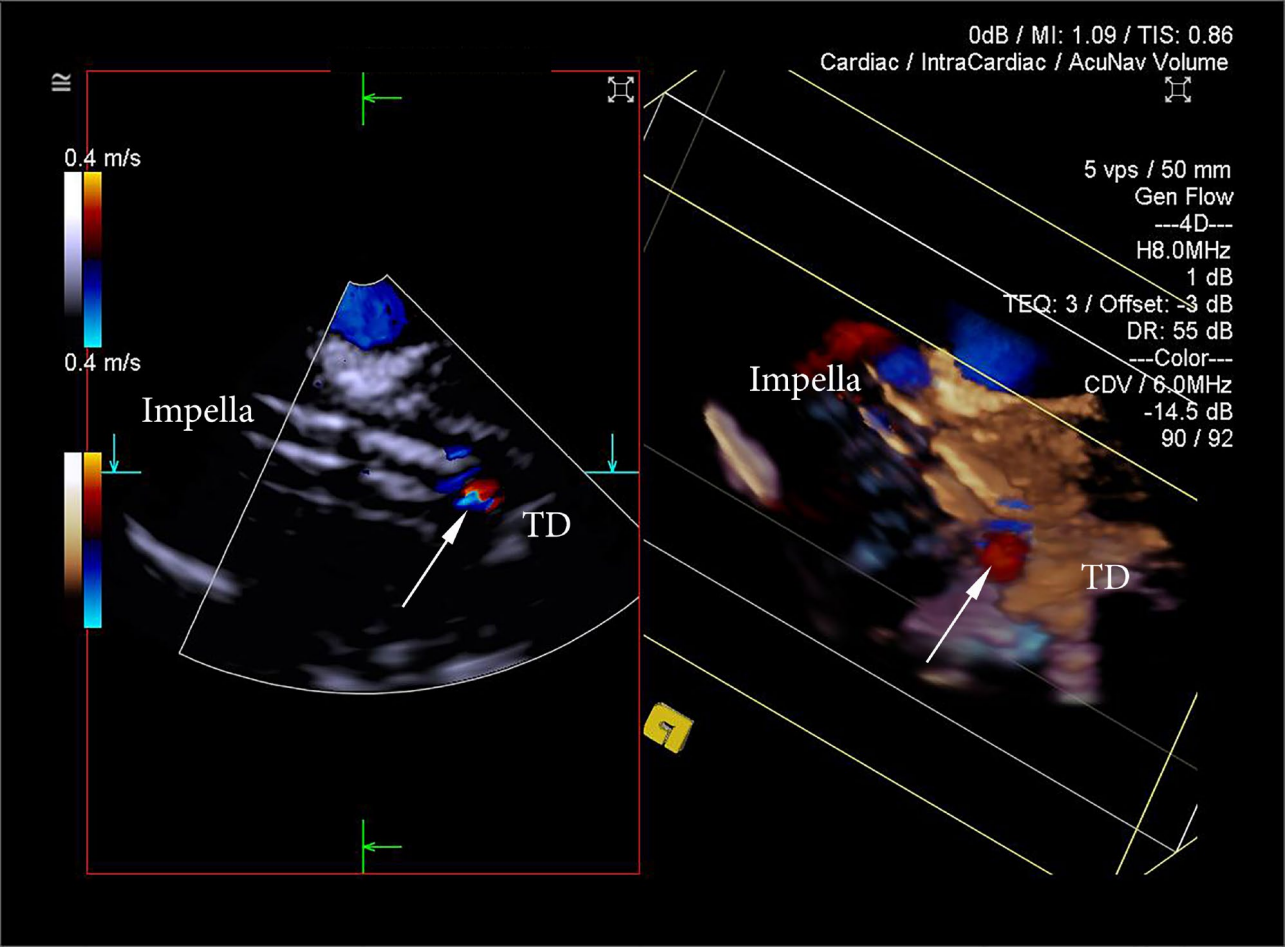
3D ICE Colour Doppler. The colour gain was significantly reduced to minimise interference from intraventricular flows and turbulence associated with Impella. The inflow was identified as an area near tear-drop (TD) on grey-scale imaging and confirmed with present inflow by Colour Doppler (white arrow).
